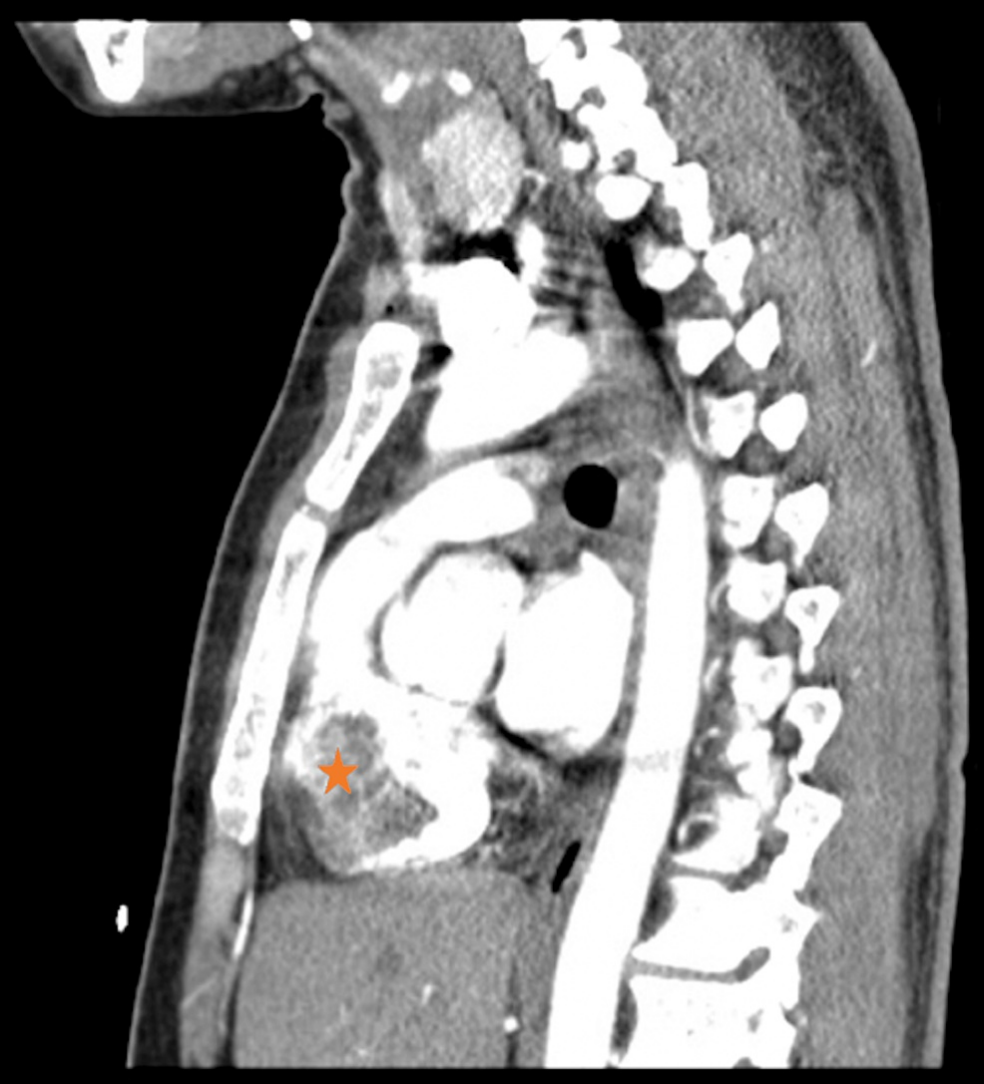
Computed tomography of the chest with contrast (sagittal view) showing a hypoenhancing defect within the right ventricle (asterisk).
